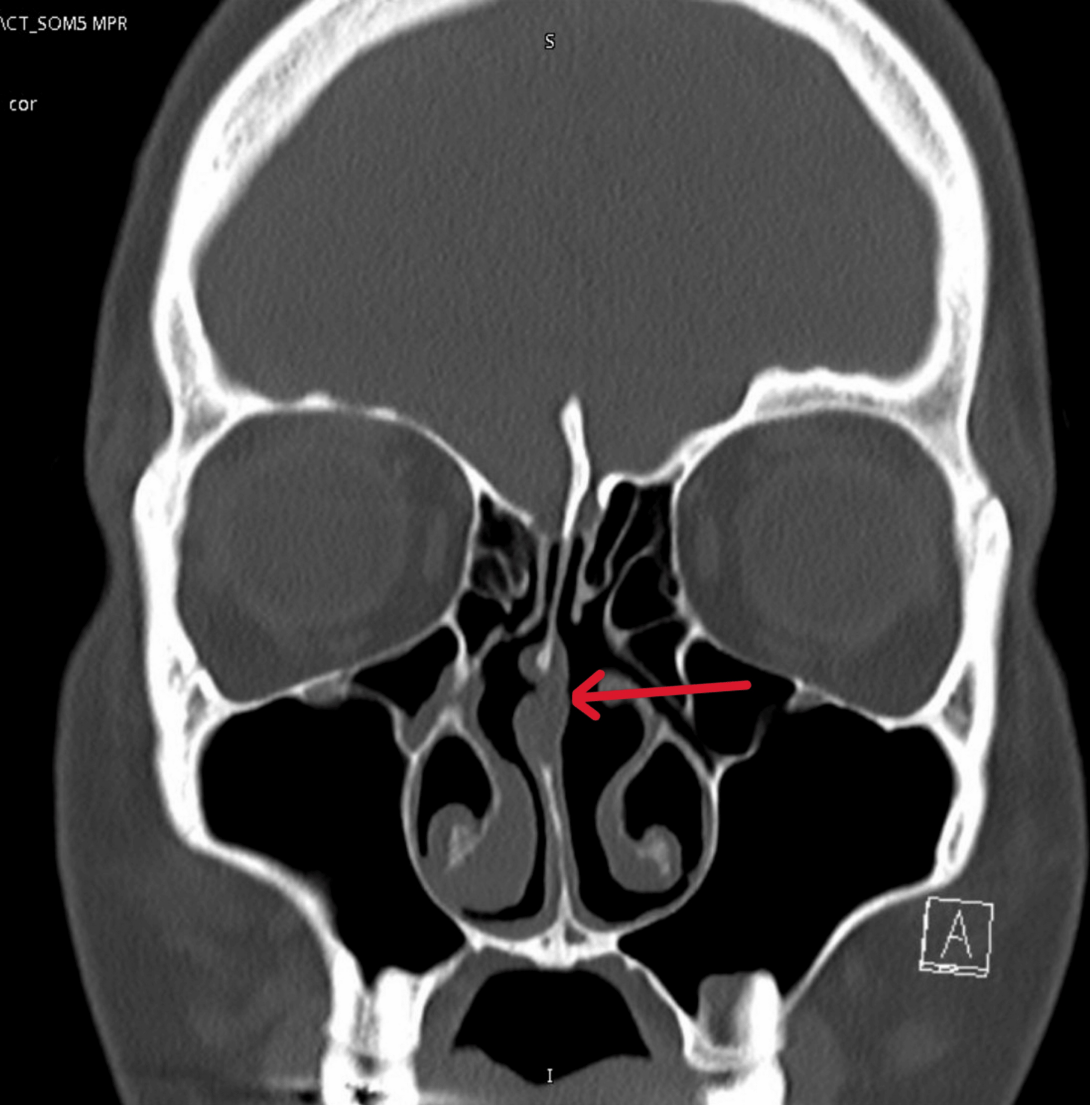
Postoperative CT scan. Note the missing cartilage (red arrow)
